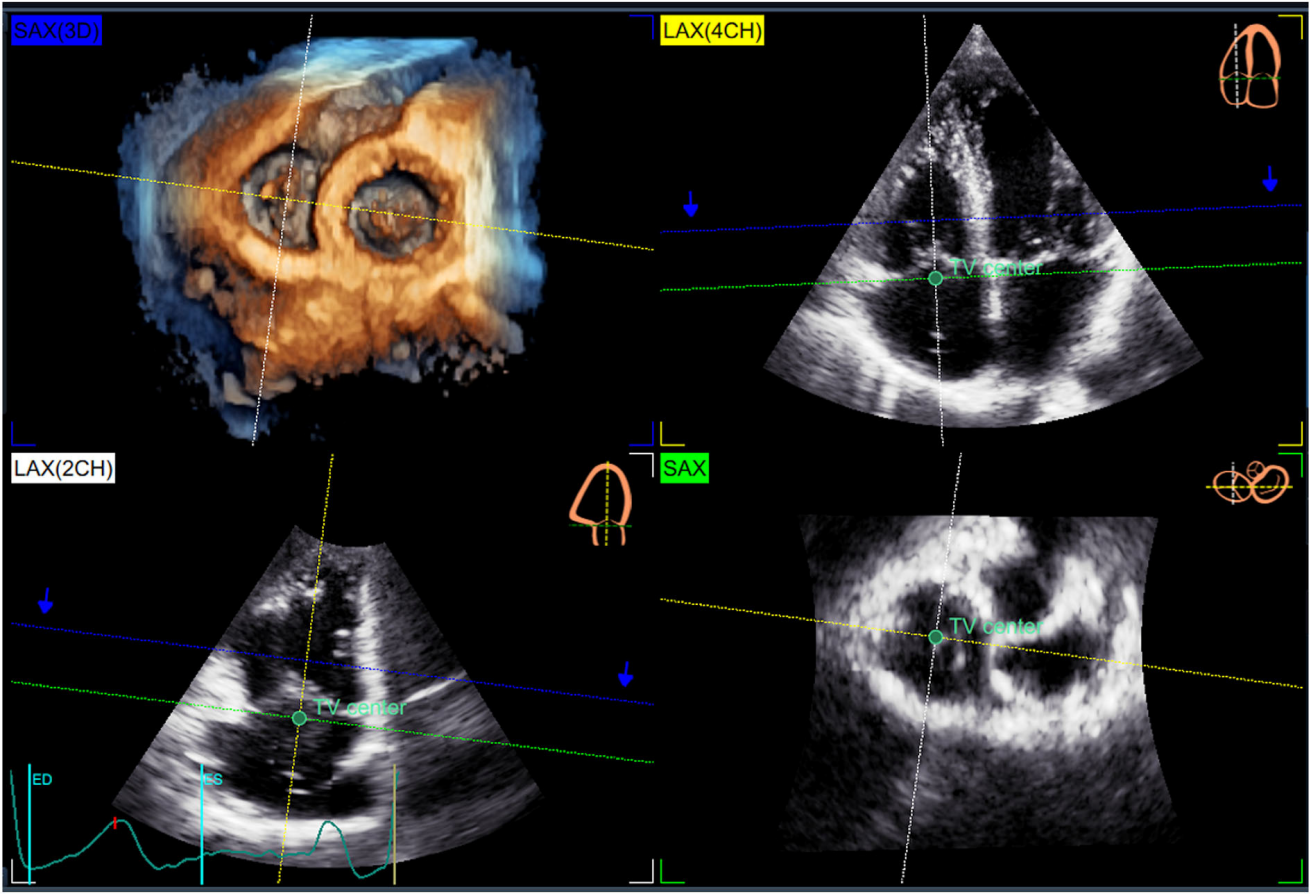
Automatic slicing of the three-dimensional echocardiography dataset to start the quantitative analysis of the tricuspid annulus. The 3DE data set [SAX(3D) panel] is automatically sliced to obtain 3 cut planes corresponding to the apical 4-chamber view [LAX(4CH) panel], its orthogonal longitudinal cut-plan [LAX(2CH) panel], and a transversal cut plane (SAX Panel). The transverse cut plane is positioned at the hinge points of the tricuspid valve leaflets with the annulus (green broken dashed line), and the longitudinal cut planes (yellow and white broken dashed lines) positioned to intersect the center of the TA (green dot) and the right ventricular apex.
